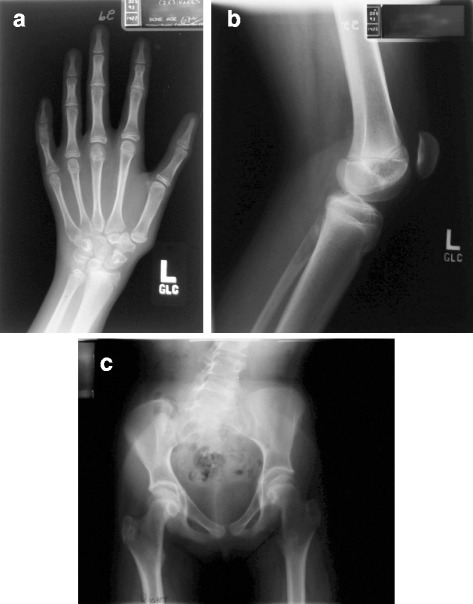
a Radiograph of the left hand for bone age: The distal phalanx of each digit is small and narrow. Shortening of the middle phalanx of digits 2 and 5. Slender metacarpals 4 and 5 and short 5th metacarpal. The margins of the carpal bones are more angular than smooth. b Lateral radiograph of the Left Knee: The patellar height ratio, measured length of the patellar tendon divided by length of the patella is 1.7, normal is 1.5. Typically increased PHR is associated with increased risk of patella dislocation. c AP standing pelvis radiograph: Pelvic tilt demonstrating a leg length discrepancy. The iliac bones are narrow. Bilateral Coxa Valga is present. Acetabular coverage is good. Dysplasia of the femoral epiphysis (flat and small)
